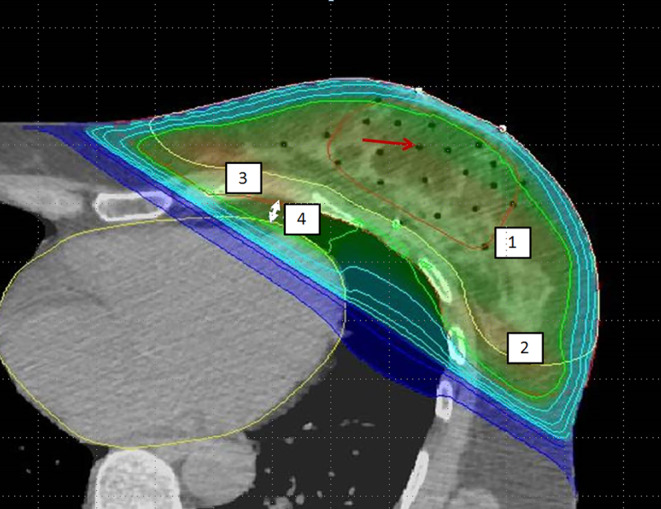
Tangential field treatment plan in a brachytherapy planning CT: *1 *demarks the brachytherapy PTV (*red*); *2* the mammary gland; *3* the EBRT PTV, the *red arrow* shows the air within a brachytherapy single leader catheter; *4* the measure of the PTV–heart distance (*white arrows*) for WB
